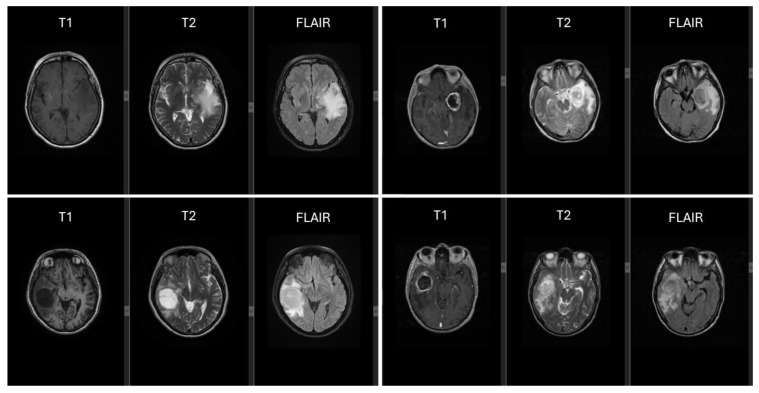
MRI sequences, including T1, T2, and FLAIR, highlight a variety of tumor volumes, locations, and lateralities, each exhibiting peritumoral edema of varying shapes and sizes.
